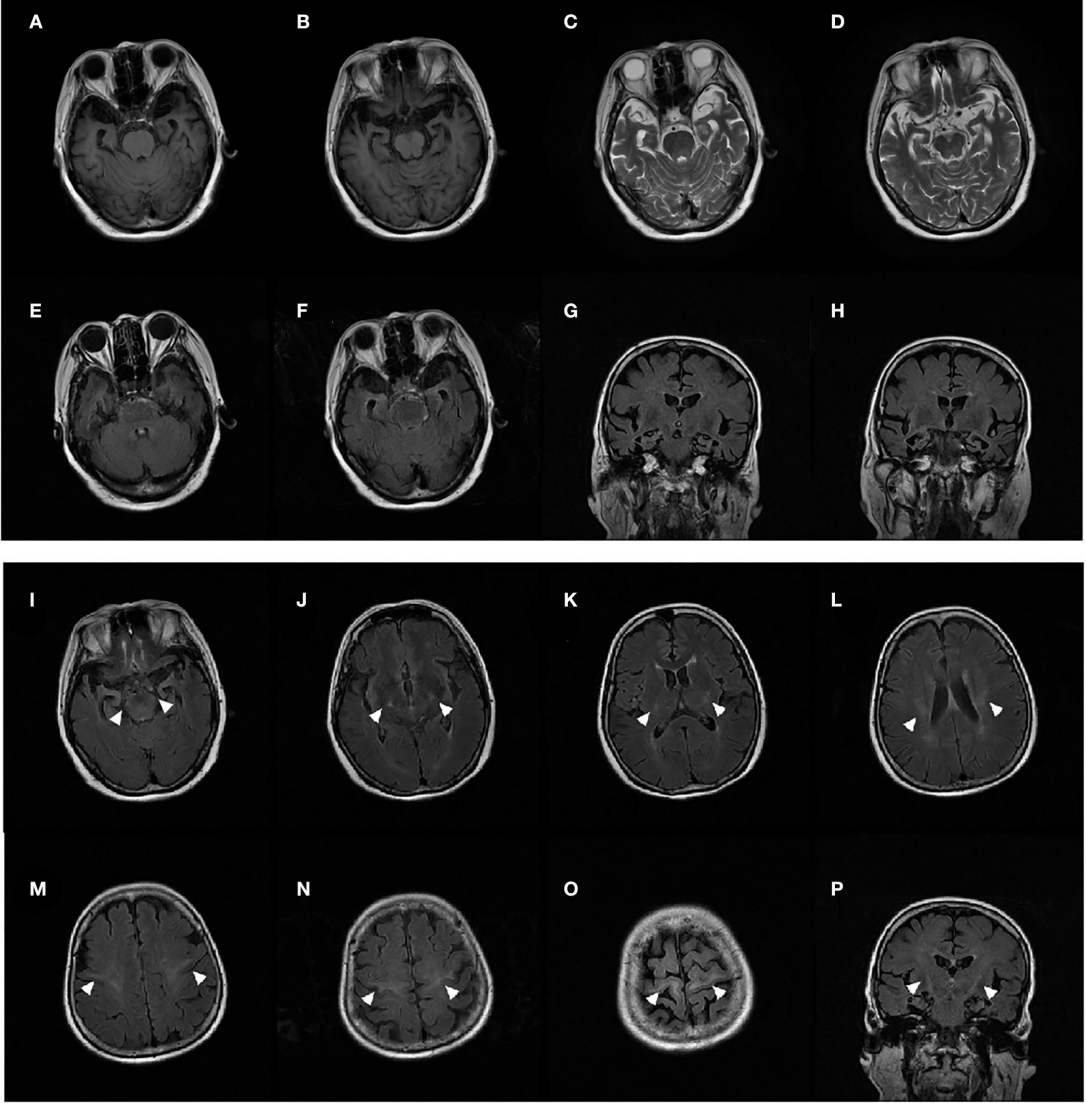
MRI of patient 1 showed significant bilateral temporal lobe atrophy (A–H). T2-weighted fluid-attenuated inversion recovery (FLAIR) coronal and axial MRI displayed bilateral signal hyperintensities along the corticospinal tracts in the primary motor cortex, centrum semiovale, posterior limb of the internal capsule, and the cerebral peduncle (I–P) (arrows).
